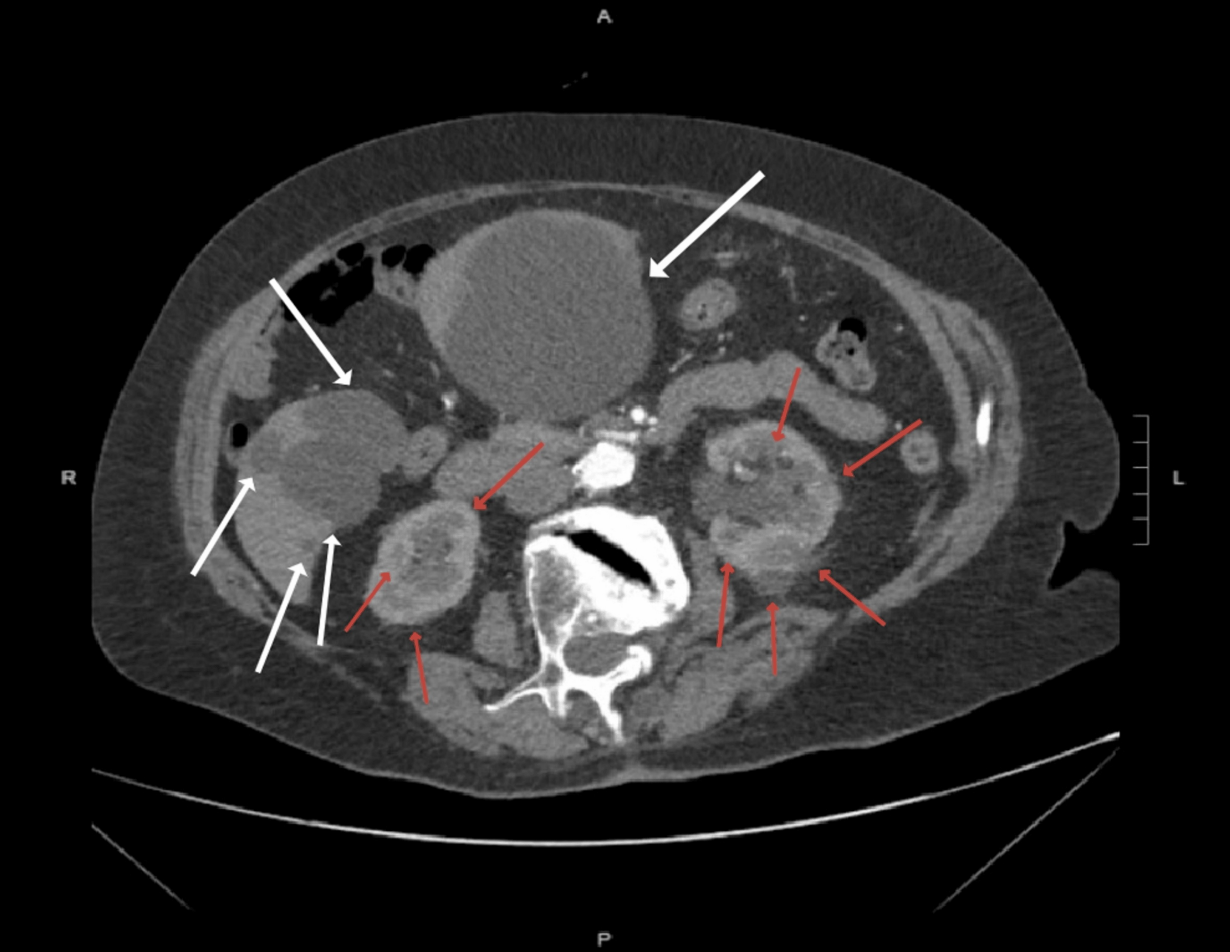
The CT image of the abdomen in the axial plane showing numerous bilateral renal cysts and multiple hepatic duplication cysts. Red arrows: Renal cysts, White arrows: Hepatic duplication cysts
